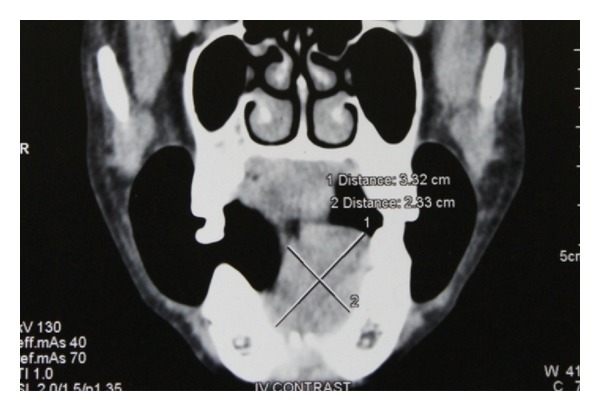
Axial CT shows dense mass of 3.32 cmm 2.33 cmm in the floor of the mouth.
